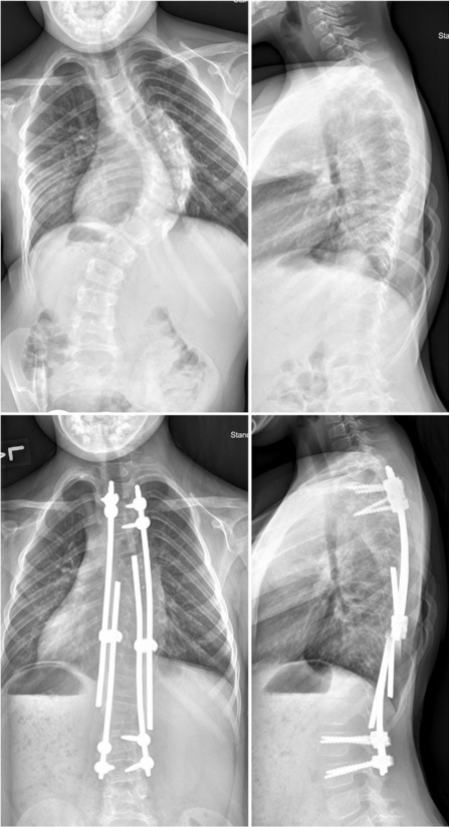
Radiographs of a patient with early onset scoliosis, preoperative (*top*) and after implantation of growing rod construct (*bottom*). (*Left*) Posterior-anterior view. (*Right*) Sagittal view
